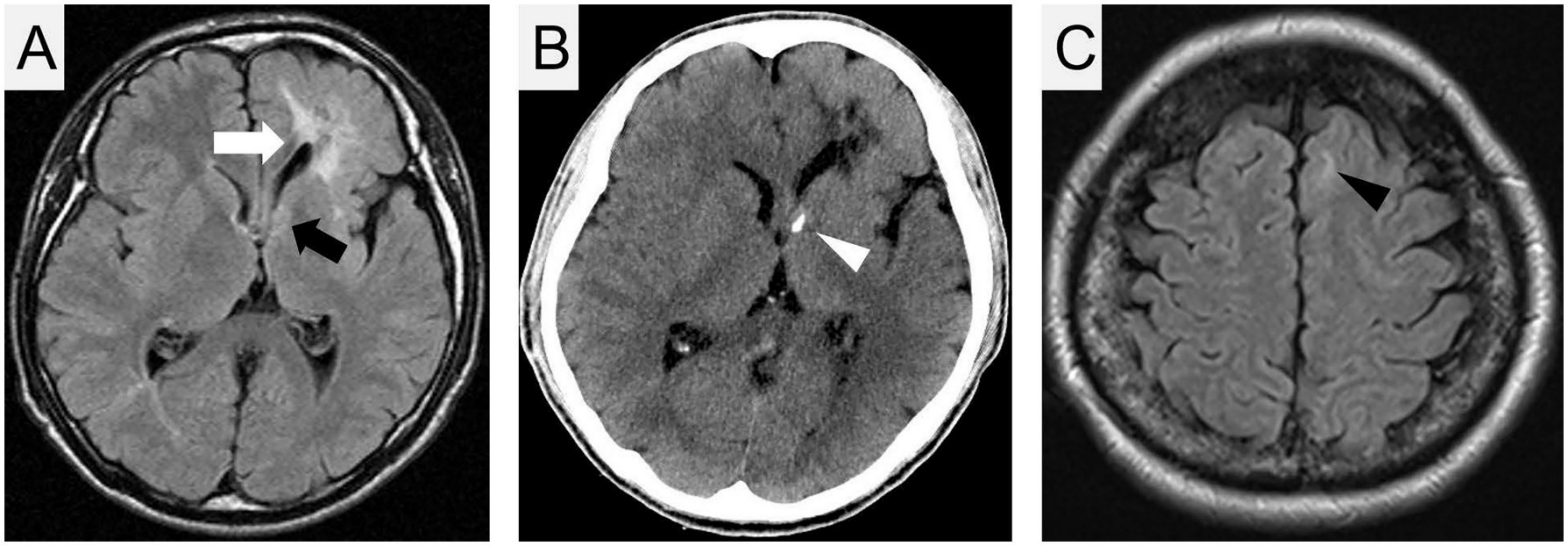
Brain imaging. Axial magnetic resonance imaging (MRI; T2 fluid-attenuated inversion recovery) (A) and computed tomography (CT) imaging (B) of patient 3. The high-intensity area near the left lateral ventricle represents cortical dysplasia (tubers and radial migration lines; white arrow). The high-intensity area, which was observed in MRI (black arrow) with calcification in CT (white arrowhead) near the left Monro foramen, represents a subependymal nodule. (C) MRI (T2 fluid-attenuated inversion recovery) of patient 1. The high-intensity area in the left frontal lobe represents a cortical tuber (black arrowhead).
